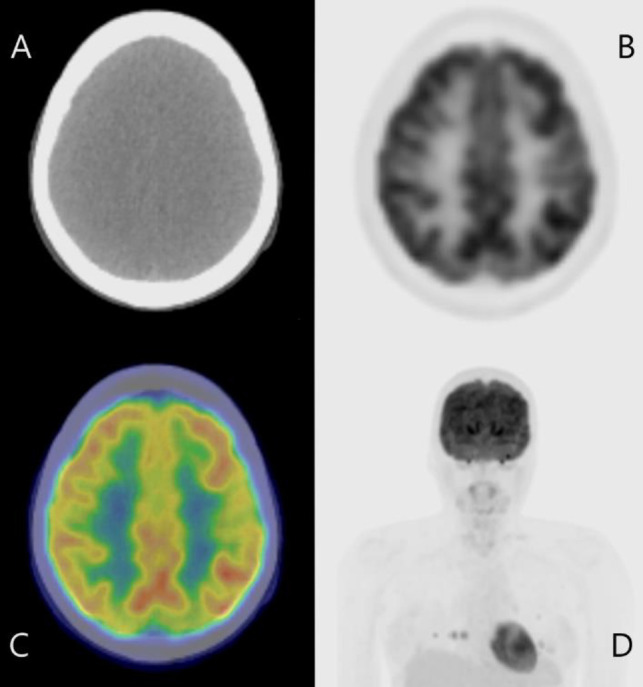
FDG-PET before radioactive iodine therapy (RAIT) showed no intracranial accumulation (A: CT for attenuation correction, B: FDG-PET (SUV range: 0-12), C: fusion image of CT and FDG-PET, D: anterior image of maximum intensity projection of FDG PET)
